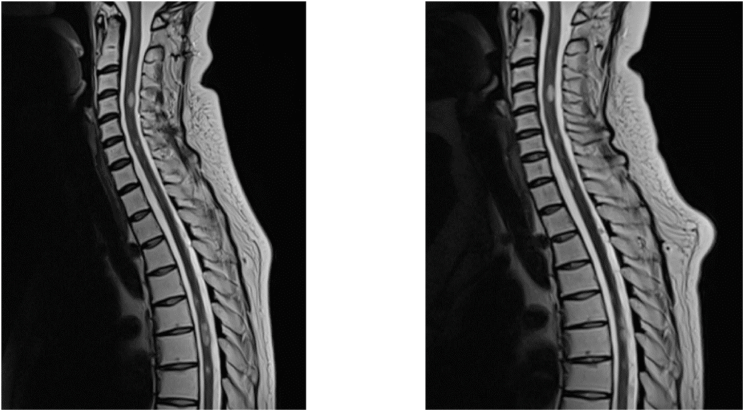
Intramedullary metastases leading to paraparesis in the patient. Sagittal T2 MRI of the cervical and upper thoracic spine before (left) and after radiotherapy (right). A slight reduction in metastasis size was achieved at the Th6 level.
